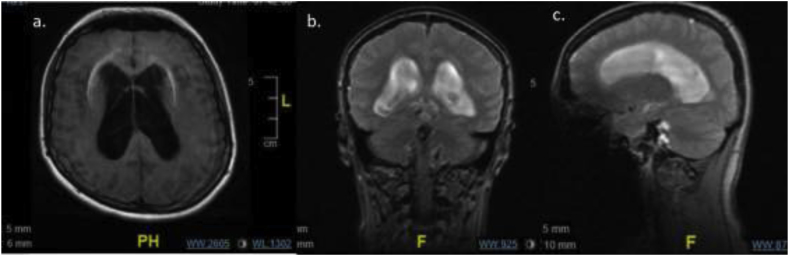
MRI on presentation (day 0), ventriculomegaly is seen on all three views: T1 flair axial (a), T2 coronal (b), T2 saggital (c). Lack of ventriculomegaly of the fourth ventricle and any obstructive mass is best visualized on saggital view (c).
